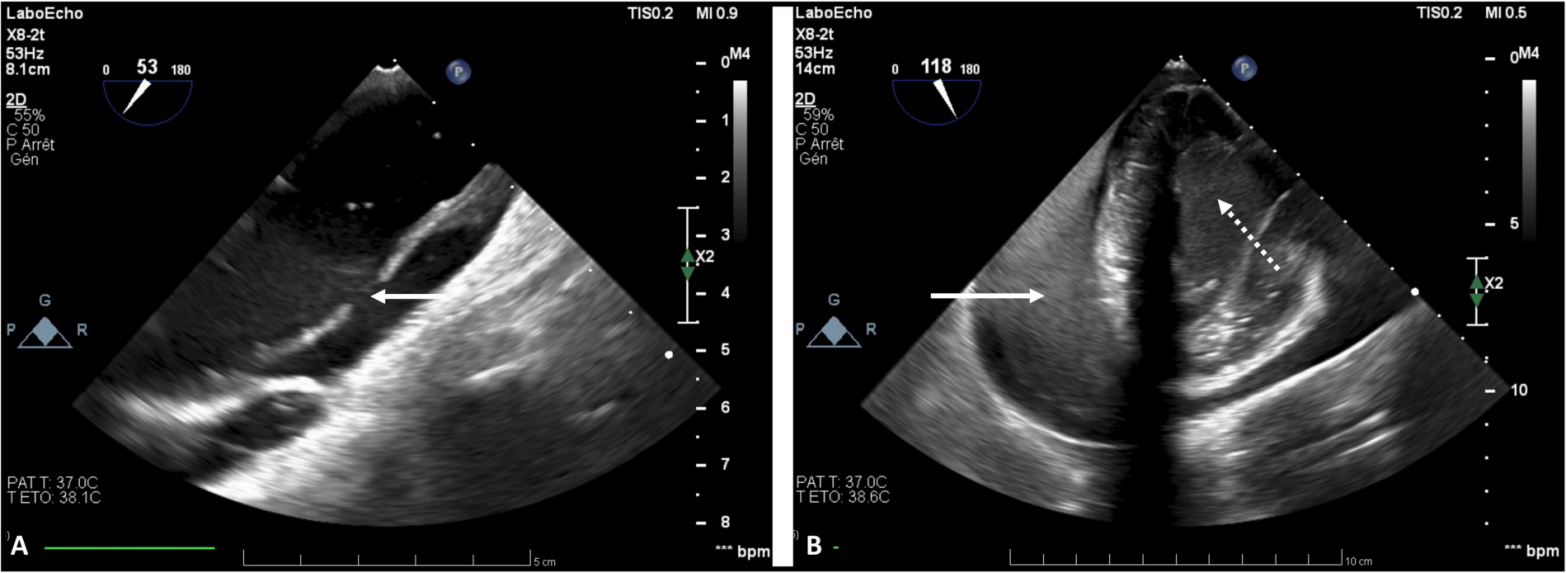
Transesophageal echocardiography while on VA-ECMO support showing Stanford A type aortic dissection of the ascending aorta [ (A) white solid line arrow], cardiac tamponade [ (B) white solid line arrow], and spontaneous echo contrast in the left ventricle [ (B) white dotted line arrow].
